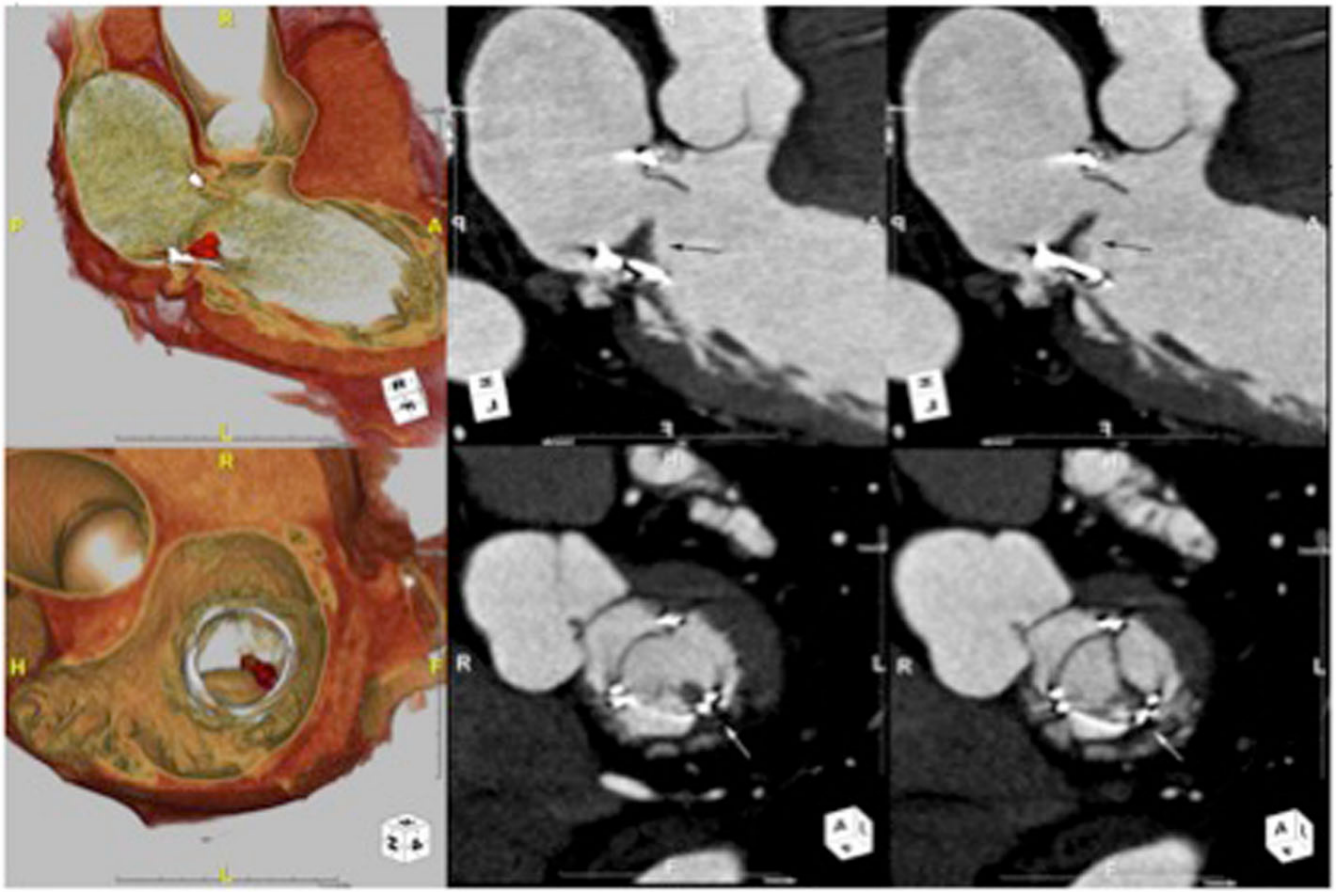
74-year-old female. 2.3 years out of mitral valve replacement. CT image demonstrated a HALT in a posteromedial single leaflet
